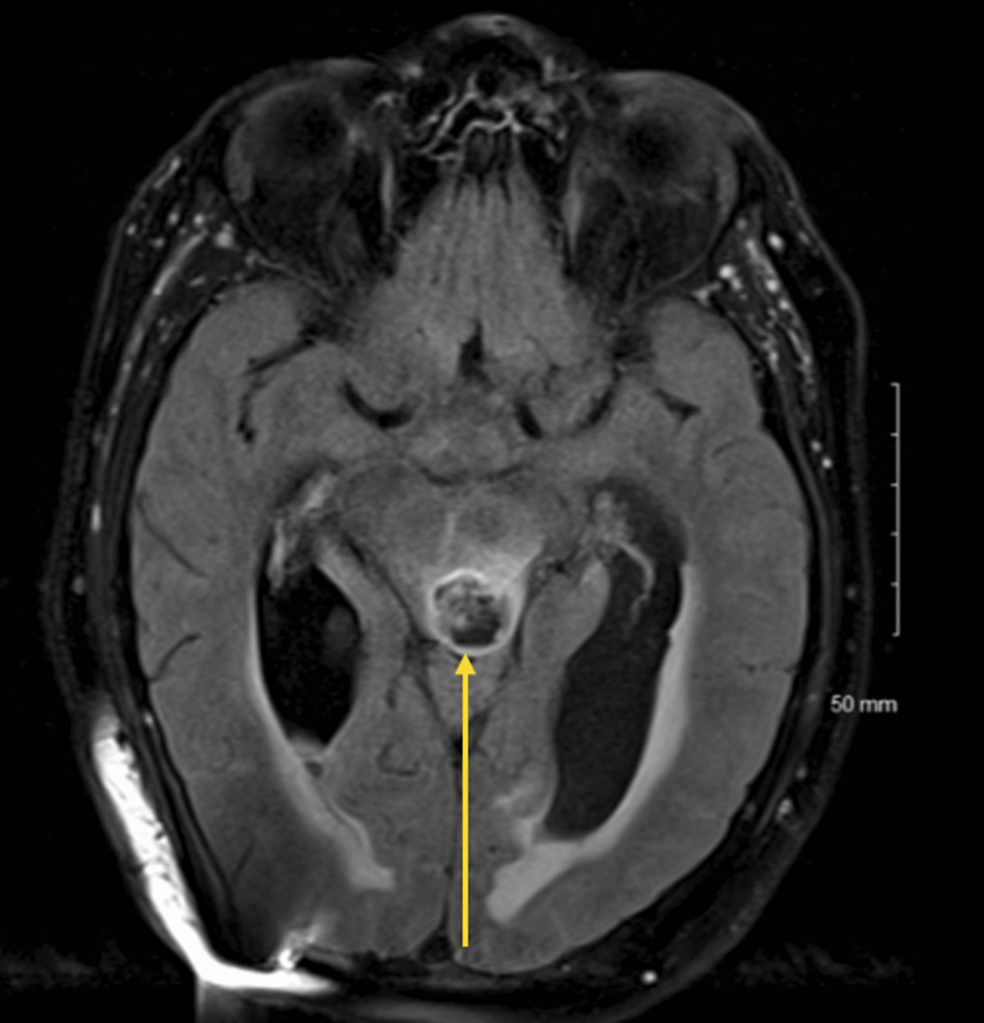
Postoperative MRI Postoperative MRI revealed an interval decrease in the volume of abnormal infiltrative hyperintense T2 signal in the brainstem. The enhancing component of the lesion has also decreased in size, with a further decrease in the size of the ventricles and interstitial edema.
